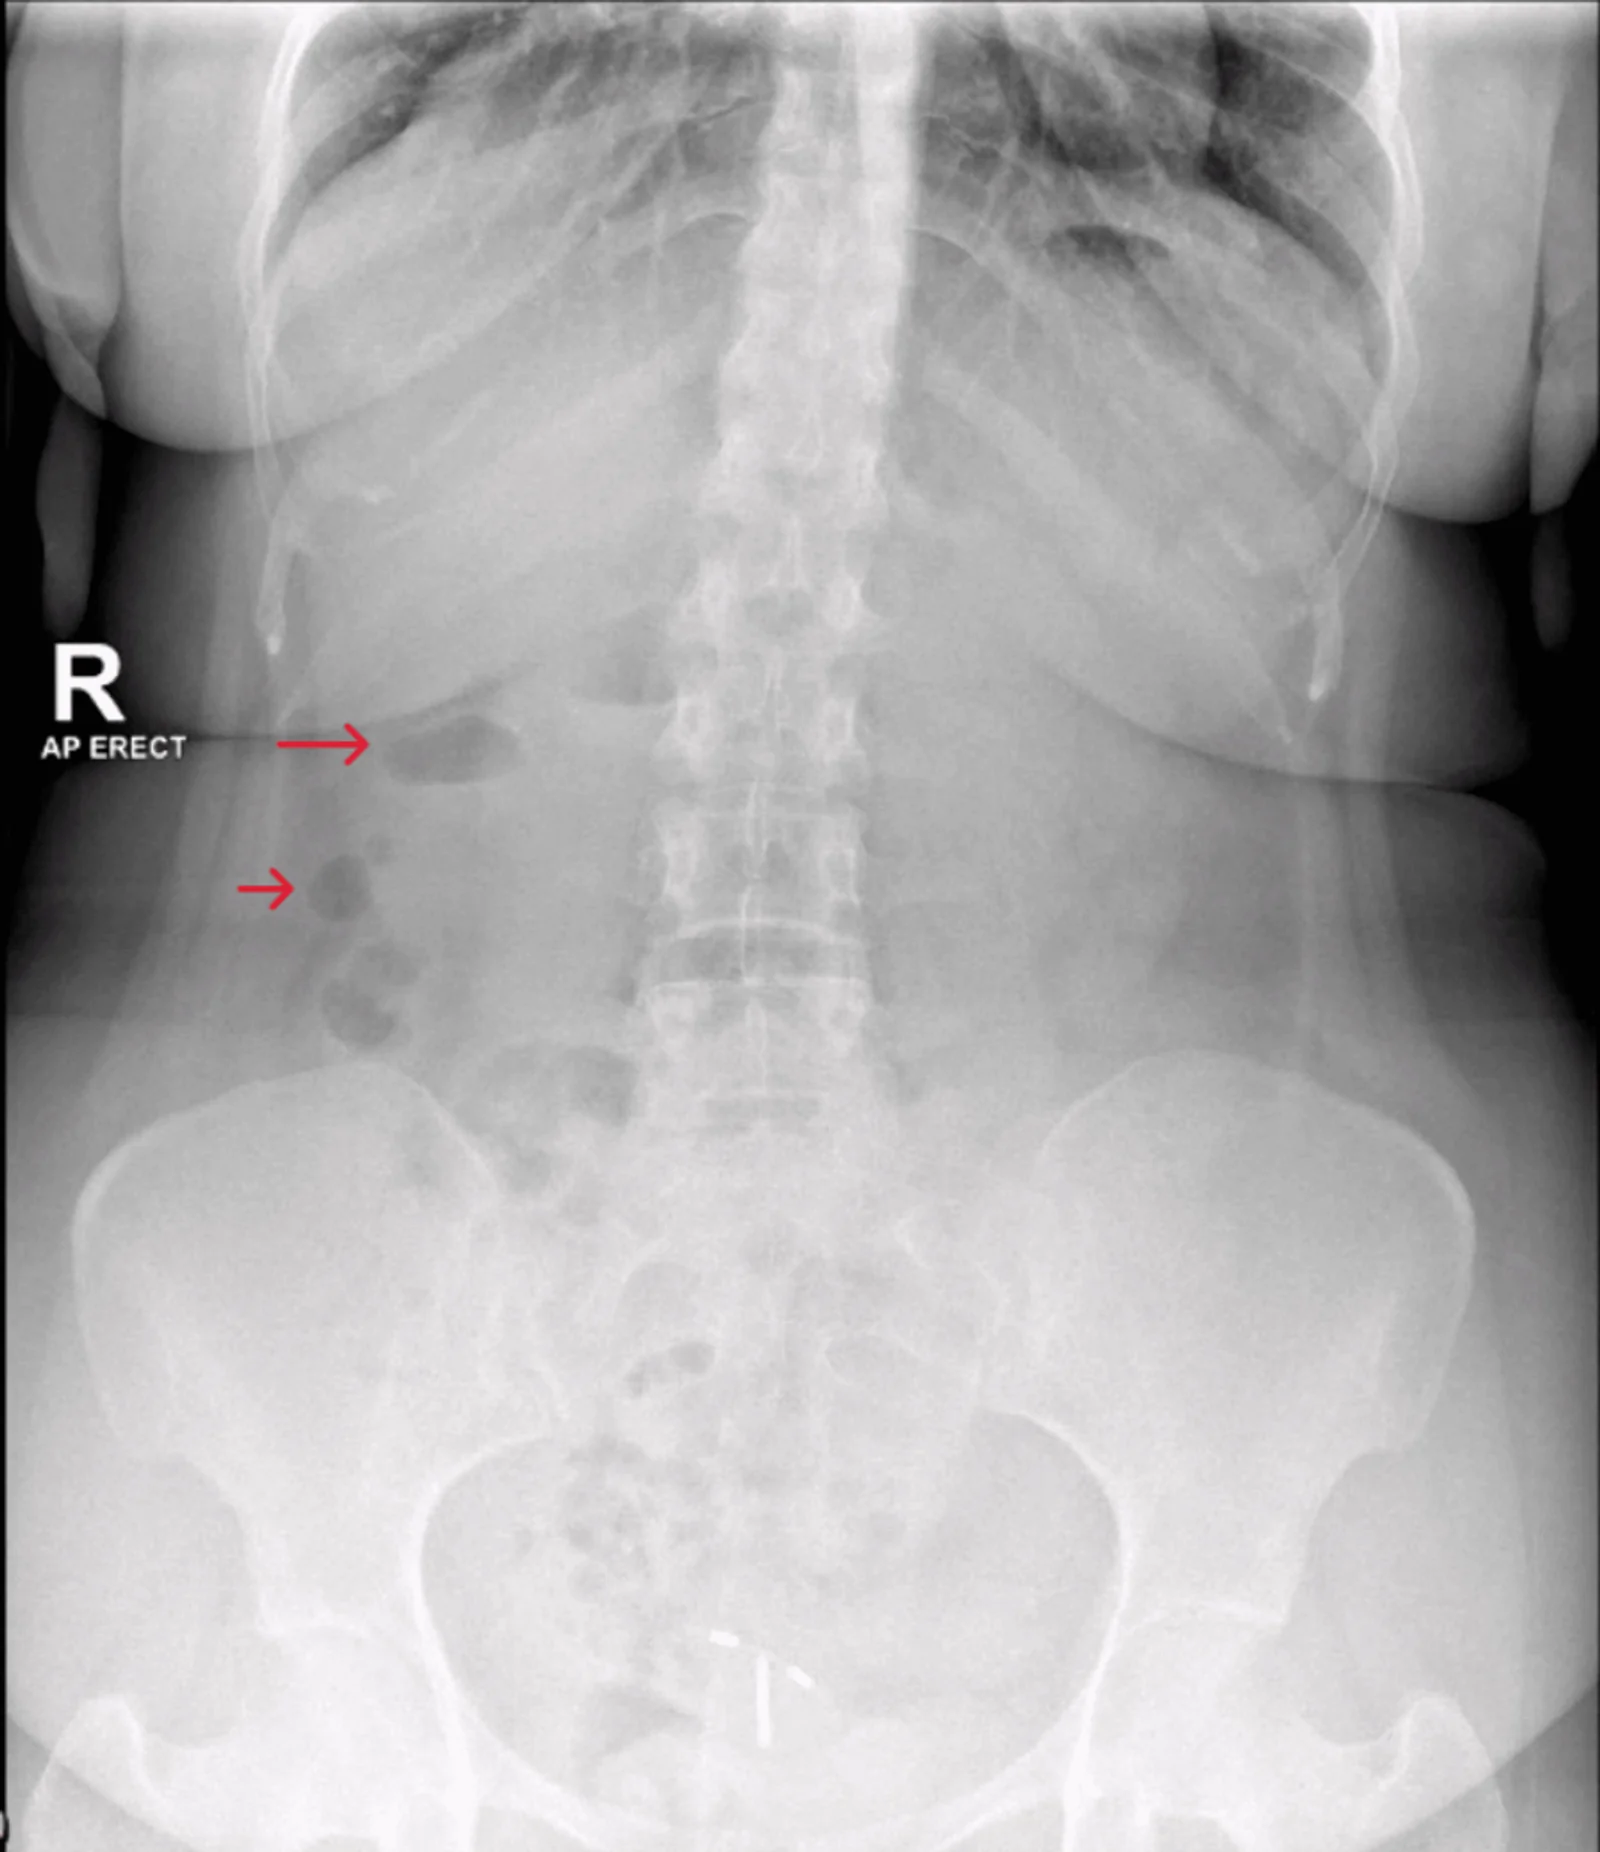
Chest and abdominal radiograph showing no evidence of bowel obstruction or pneumoperitoneum. Chest and abdominal radiograph demonstrating normal cardiac silhouette and clear lung fields. The abdominal gas pattern is unremarkable (as indicated by the red arrows) with no air-fluid levels or subdiaphragmatic free air. No radiopaque pathological shadows are seen. An IUCD is incidentally noted in situ. IUCD: intrauterine contraceptive device.
